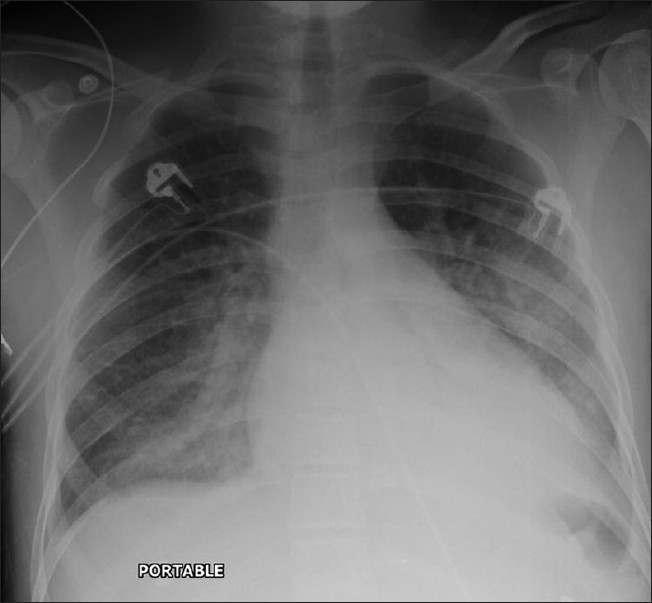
Anterioposterior chest radiograph on a 43-year-old man treated with highly active antiretroviral therapy for over 8 years who presented with increasing shortness of breath over the past 3 months that had suddenly worsened, prompting hospital admission. The chest X-ray shows features of pulmonary edema. Subsequent investigations revealed congestive cardiomyopathy
